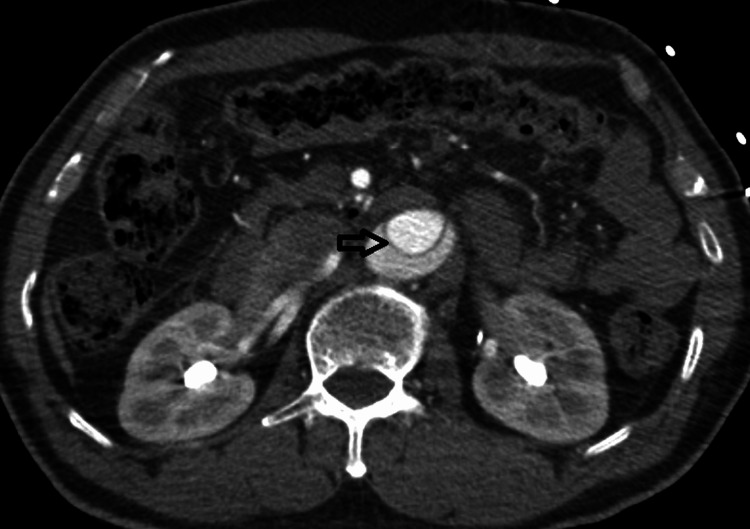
Axial CT angiography image of abdominal aorta showing linear hypodense structures within the false lumen (black arrow) with similar attenuation as the intimal flap (Cobweb sign).
